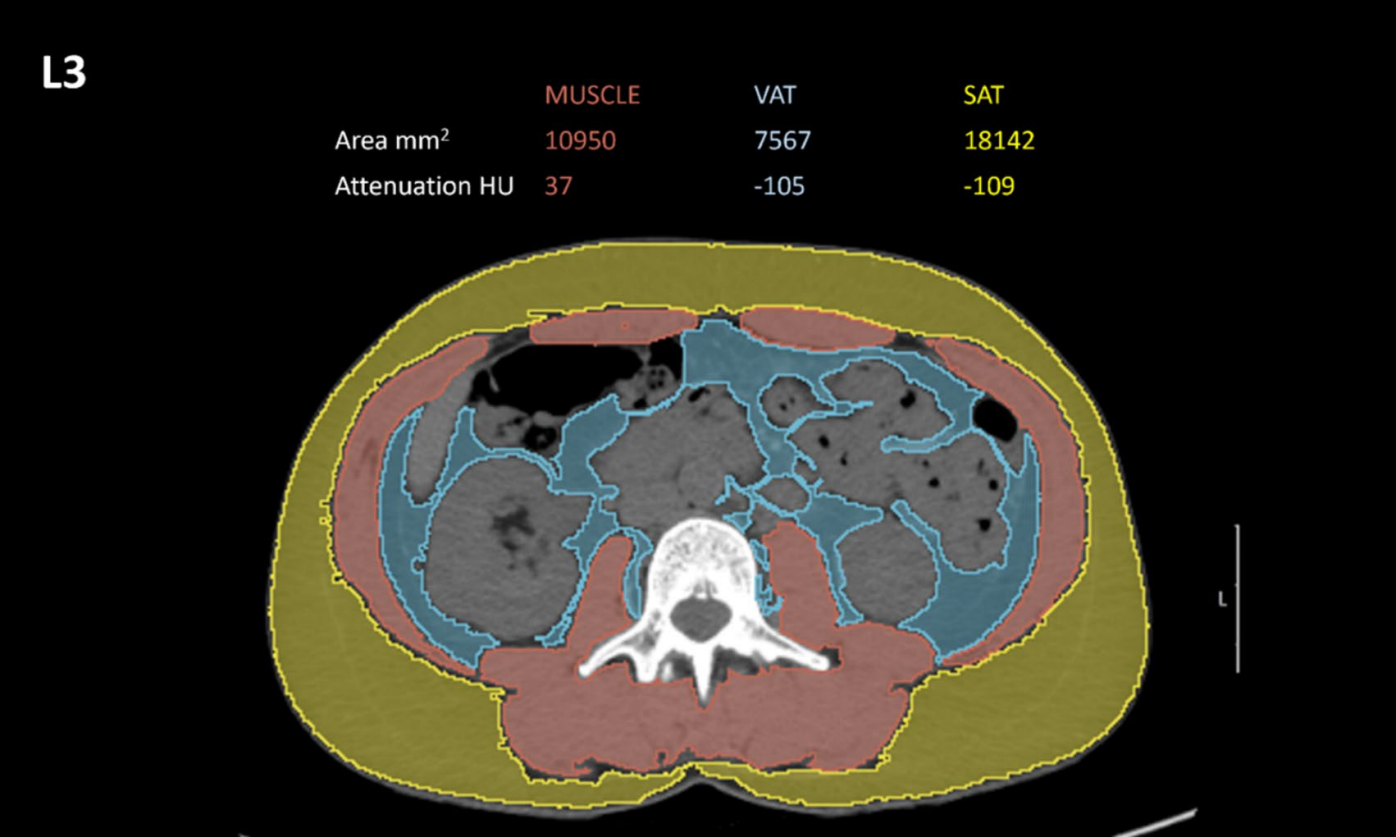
Axial CT images obtained at L3 level in 49-year-old woman which was used to determine skeletal muscle area (SMA). SMA measured 109.5 cm^2^. Blue indicates automated segmentation of visceral adipose tissue (VAT), and yellow denotes automated segmentation of subcutaneous adipose tissue (SAT)
